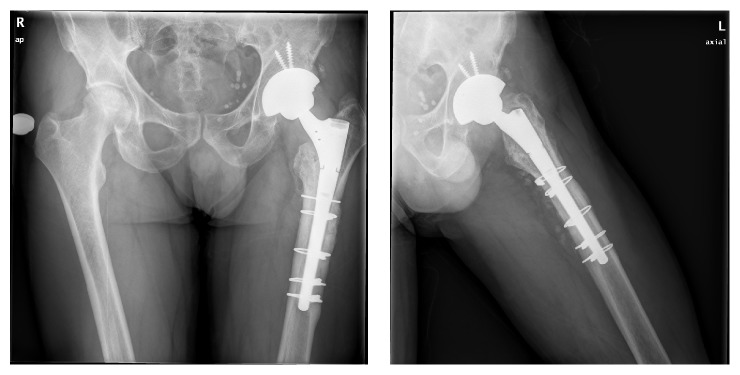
Plain radiographs of the pelvis showing a straight Revitan revision prosthesis (Zimmer, Warsaw, IN) and a TMT revision cup (Zimmer, Warsaw, IN) with press fit and two cancellous screws; the trochanteric osteotomy was synthetized with 4 cerclage wires.
